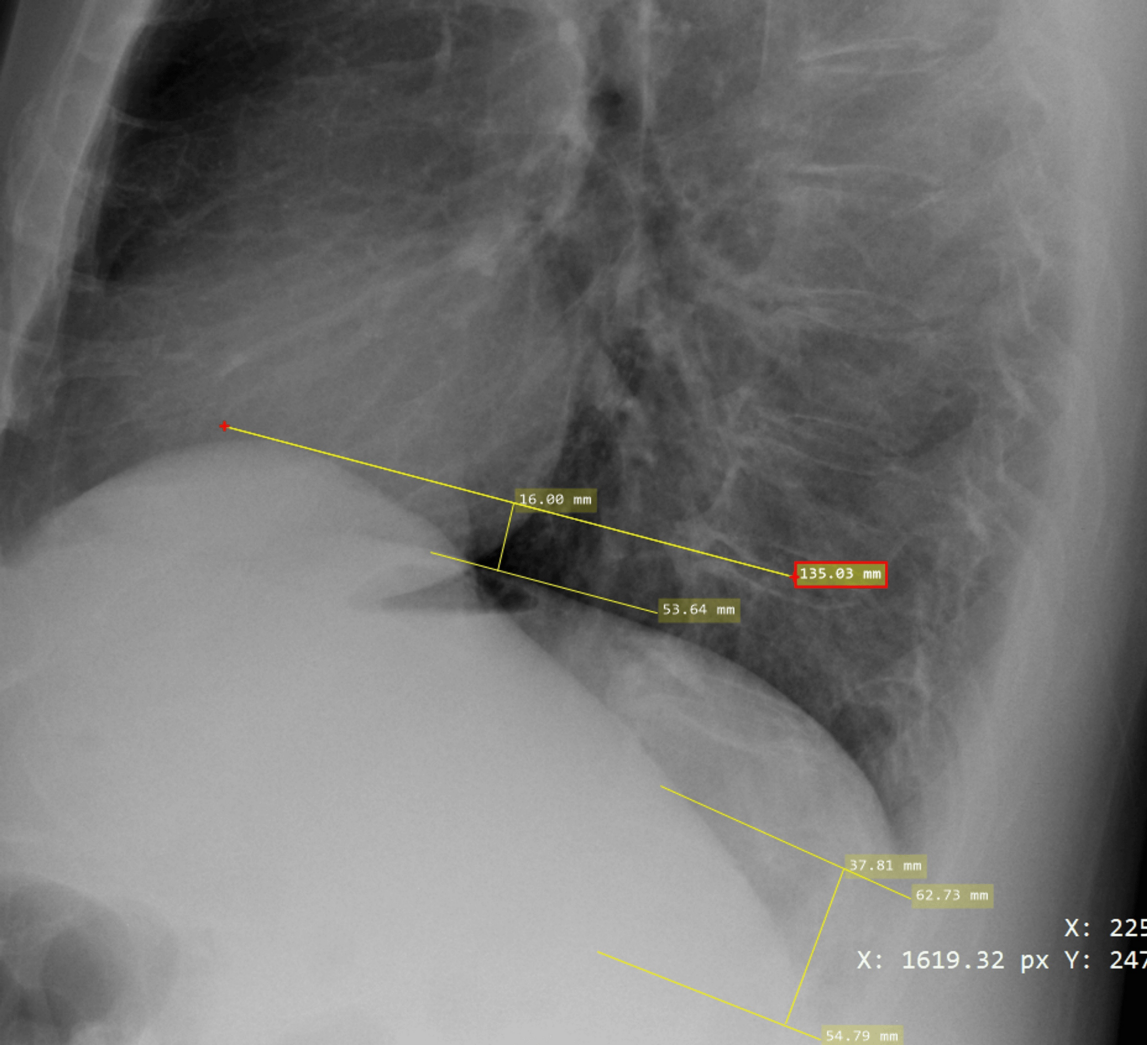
Lateral CXR projection obtained from the MIMIC-IV CXR database with evidence of DE on the right hemidiaphragm. Anterior parallel lines demonstrate the measurements of the anterior height differences, while posterior lines demonstrate the measurements of posterior height differences. Perpendicular lines represent the height distance (in cm), where the anterior measurement was 1.6 cm and posterior measurement was 3.78 cm. CXR from the MIMIC-CXR Database v2.0.0, a publicly available dataset developed by Johnson et al. at the Massachusetts Institute of Technology. Image used under a Creative Commons Attribution 4.0 International License (CC BY 4.0). Source: Johnson et al. [14] CXR, chest X-ray; DE, diaphragmatic eventration; MIMIC-IV, MIT Medical Information Mart for Intensive Care, Fourth Edition
